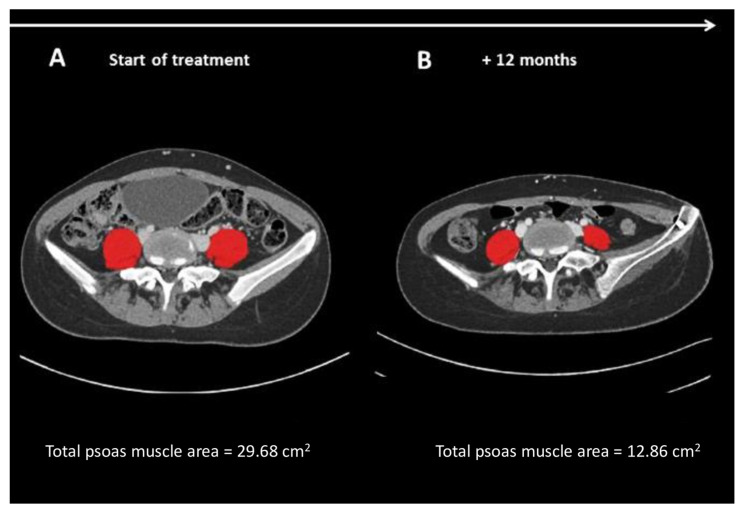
tPMA (in red color) measured from single cross-sectional abdominal CT images at the level of L4–L5 in the same patient, at diagnosis, and after 12 months of treatment.
